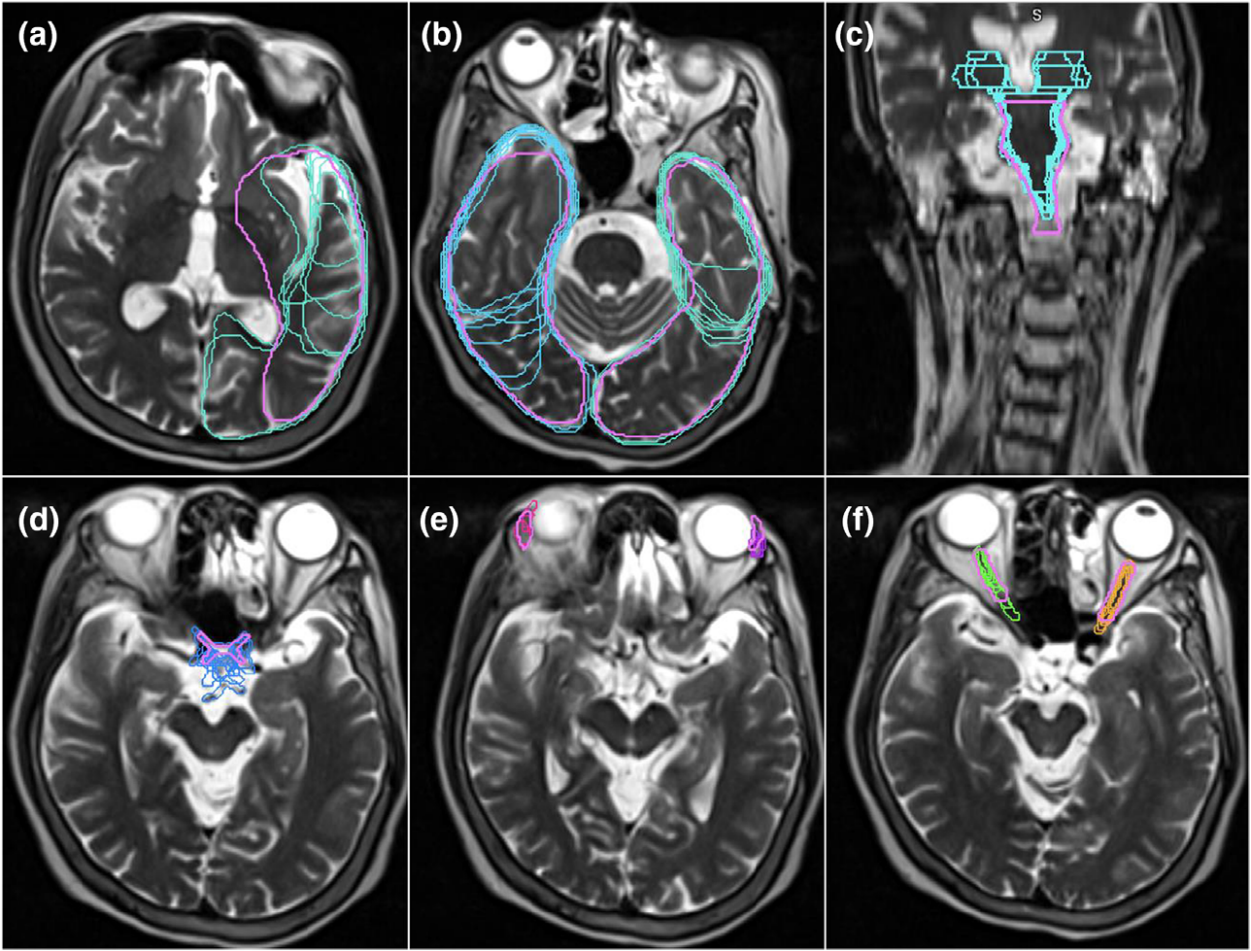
Variation in observer volumes post‐workshop. Screenshots of post‐workshop observer contours from MRI data sets in our study (pink contours indicate the radiation oncologist contoured reference volumes). (a) Left temporal lobe, (b) Left and right temporal lobes, (c) Brainstem, (d) Optic chiasm, (e) Left and right lacrimal glands, (f) Left and right optic nerves.
